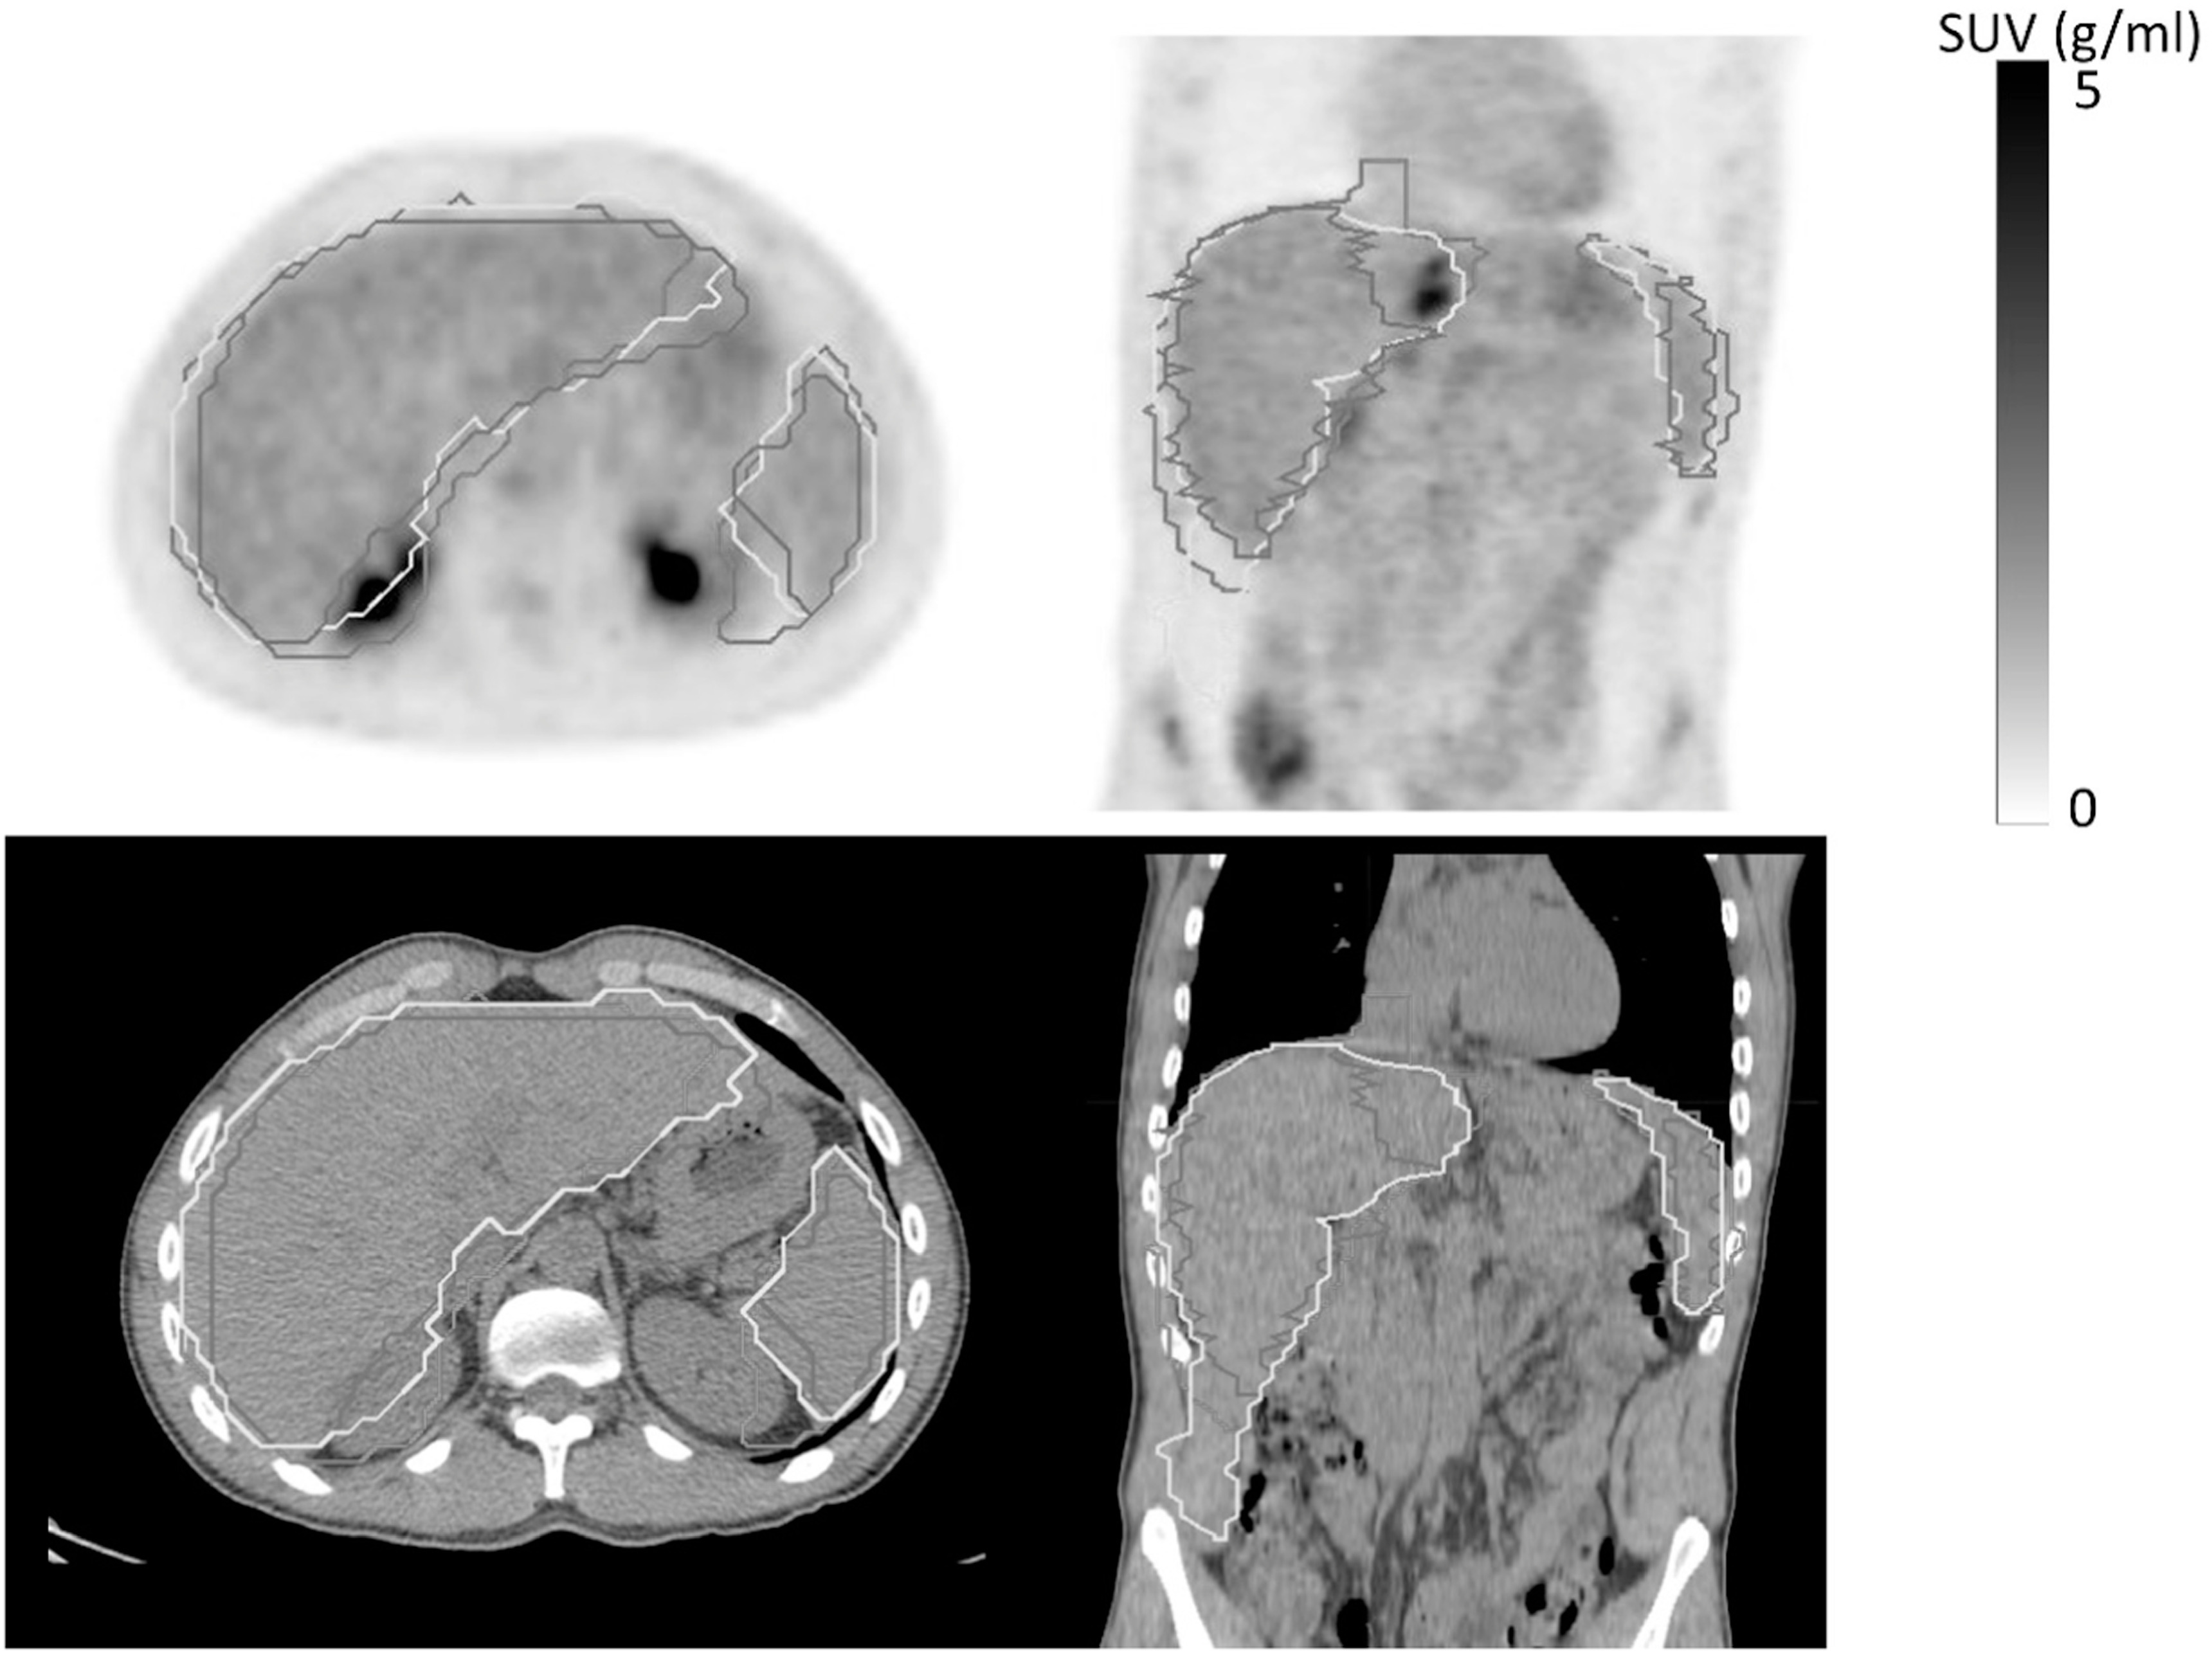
Axial (first column) and coronal (second column) view of PET (first row) and CT (second row) images a patient for which CT-DL and CT-Atlas had high DICE coefficient with PET-man but the extension of the regions to include right kidney led to high biases in SUV_max_. The brown contour represents PET-man, the yellow CT-DL and the magenta the CT-Atlas.
